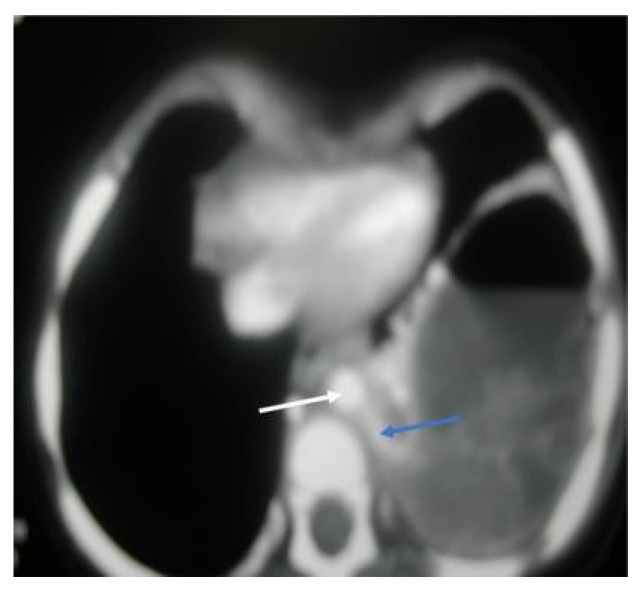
CT chest, axial window, mediastinal view: large, thick wall cavity filled with air fluid with heterogenous opacity. The white arrow shows a descending aorta, while the blue arrow confirms a large feeding vessel to the left lower lobe, a rising and descending aorta, mild compression of left upper lobe, and a mild pectus excavatum.
